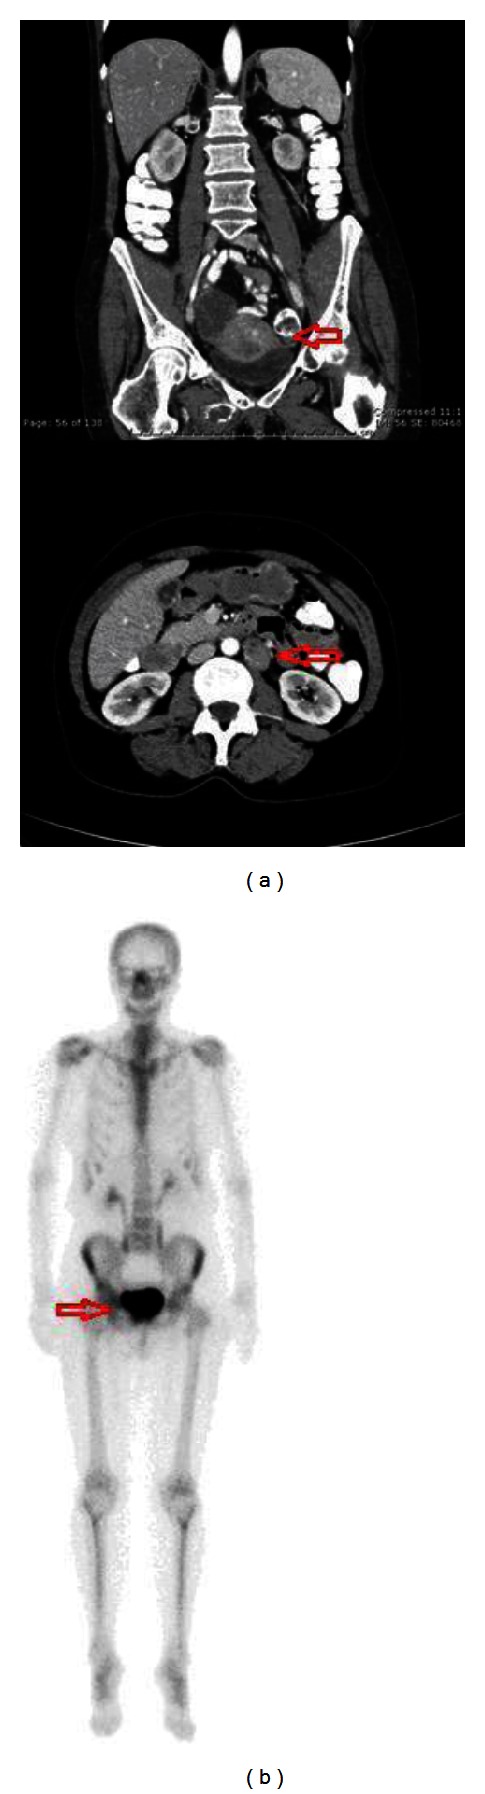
MRI (a) and bone scan (b) of uterine mass metastatic to bone.
